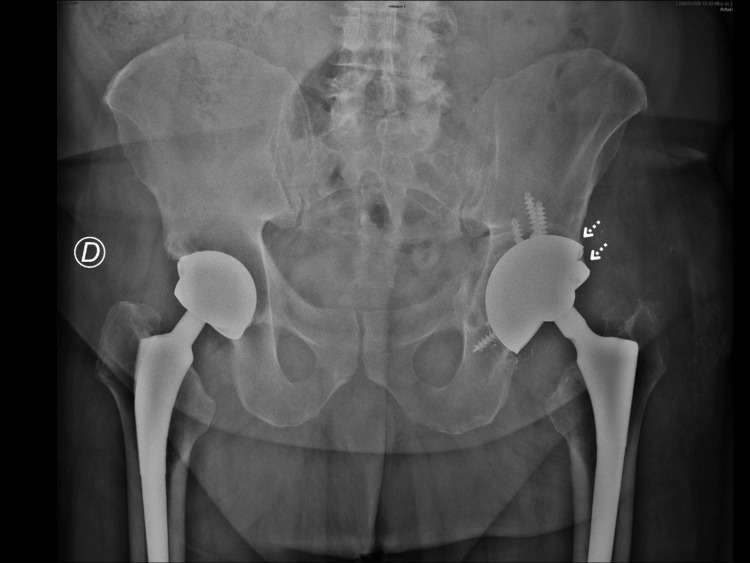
One-week postoperative AP pelvic radiograph showing stable alignment of the double-cup construct without evidence of loosening or malposition.
